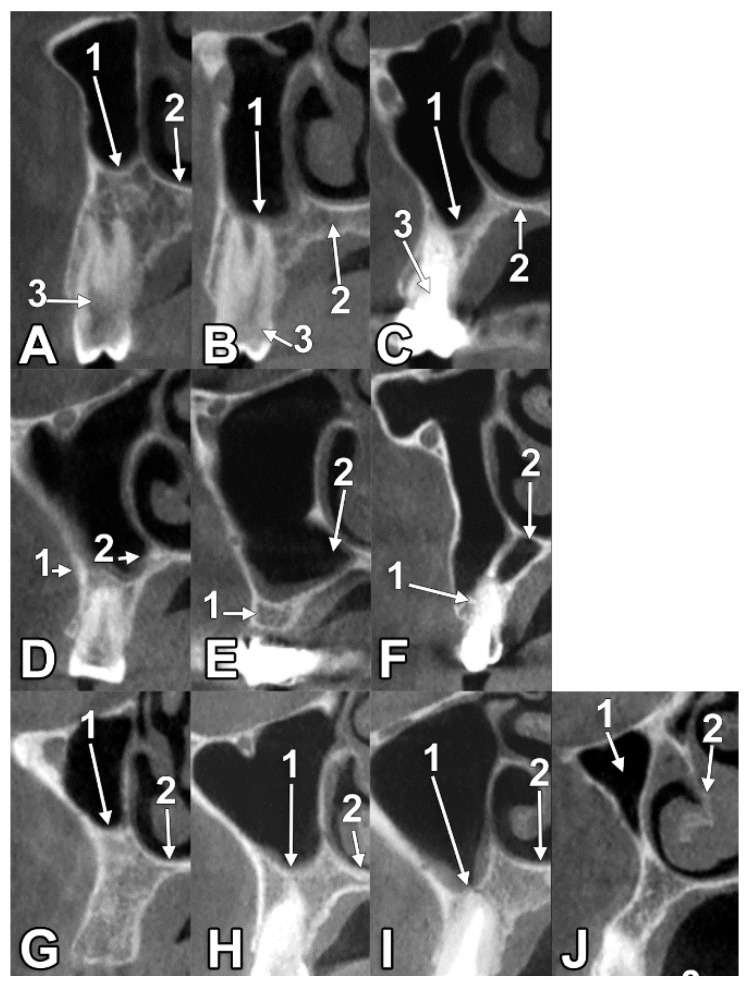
(A). Type 1a alveolar base at the right maxillary second premolar level. Orthoradial CBCT slice. 1. Antral alveolar base; 2. nasal floor; 3. second premolar. (B). Type 1b alveolar base at the left maxillary second premolar level. Orthoradial CBCT slice. 1. Antral floor; 2. palatal process of maxilla; 3. second premolar. (C). Type 1c alveolar base at the right maxillary second premolar level. Orthoradial CBCT slice. 1. Alveolar base; 2. nasal floor; 3. second premolar. (D). Type 2bO alveolar base in the region of the second left maxillary premolar. Orthoradial CBCT slice. 1. Alveolar base; 2. opened palatal recess. (E). Type 2cO alveolar base in the maxillary premolar region, right side. Orthoradial CBCT slice. 1. Alveolar ridge; 2. opened palatal recess. (F). Type 2cC alveolar base at the left maxillary second premolar level. Orthoradial CBCT slice. 1. Alveolar bone; 2. closed palatal recess. (G). Type 3a alveolar base at the right upper second premolar. Orthoradial CBCT slice. 1. Antral floor; 2. nasal floor. (H). Type 3b alveolar base at the right upper second premolar level. Orthoradial CBCT slice. 1. Antral floor; 2. nasal floor. (I). Type 3c alveolar base at the level of the left upper second premolar. Orthoradial CBCT slice. 1. Antral floor; 2. nasal floor. (J). Type 4 alveolar base at the left maxillary second premolar level. CBCT orthoradial slice. 1. Maxillary sinus; 2. inferior nasal turbinate.
